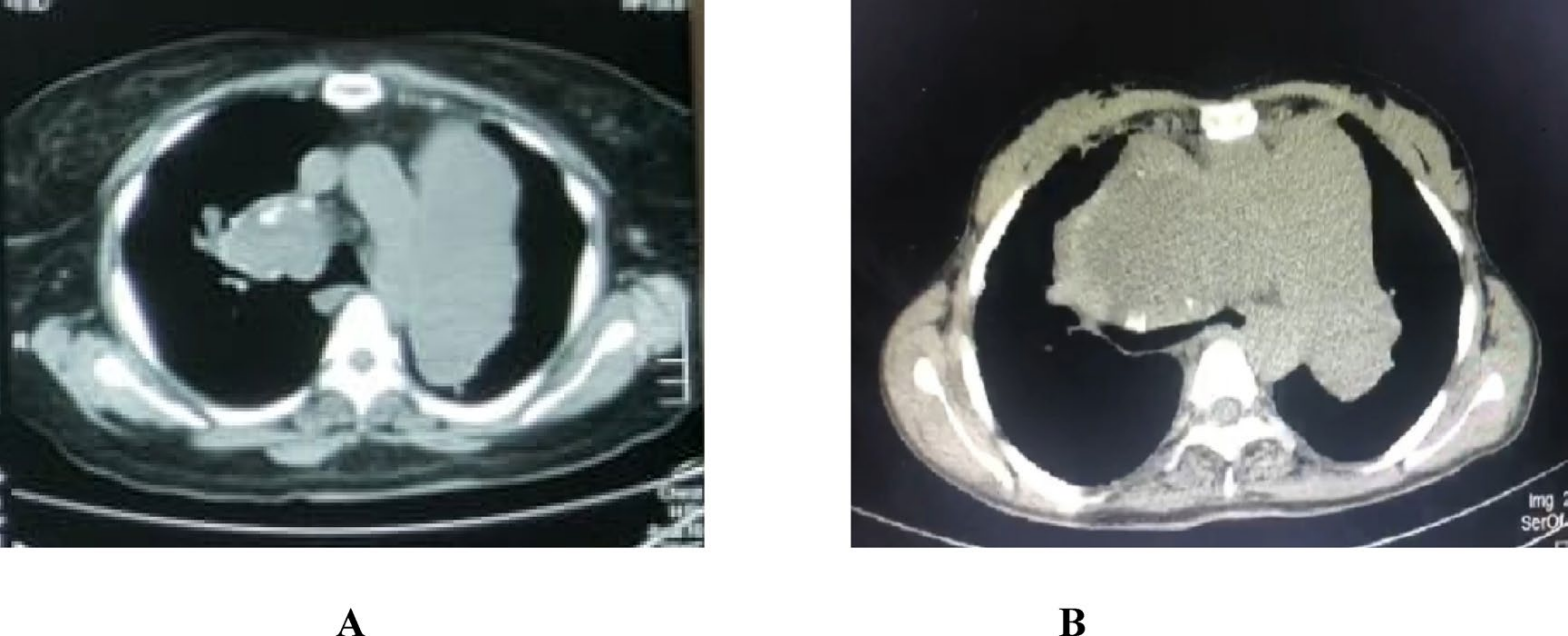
CT chest showed Pulmonary artery aneurysmal dilatation in 2 cases with Sch-PAH (A and B). Sch- PAH: Schistosomiasis-associated pulmonary arterial hypertension.
